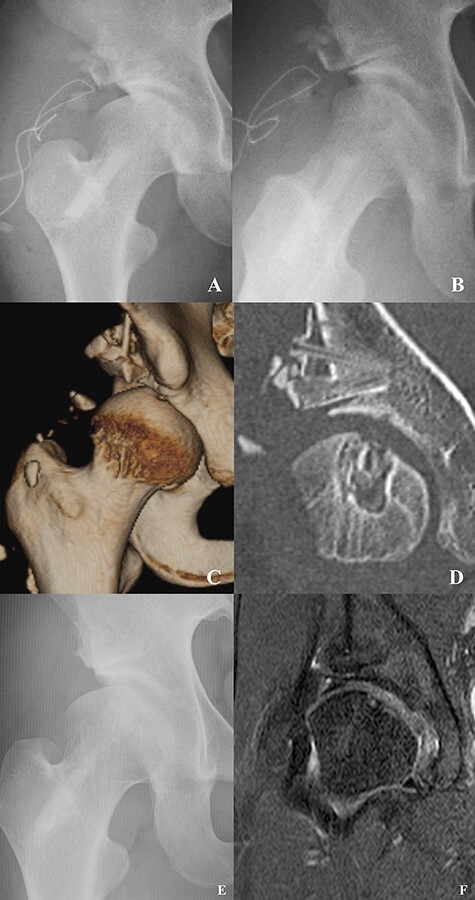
Postoperative (A) anteroposterior (AP) pelvic and (B) Dunn views showing improved lateral coverage of the acetabulum and femoral head neck offset. (C) Postoperative three-dimensional CT showing improved anterosuperior acetabular coverage. (D) Postoperative coronal CT showing bone graft incorporation and filling of the bone cyst. (E) AP pelvic view at 2 years after surgery showing the shelf graft was remodeling and no evidence of bone cyst in the femoral head. (F) The proton density fat suppression coronal view showing complete healing of the femoral head and the shelf graft was adapted.
